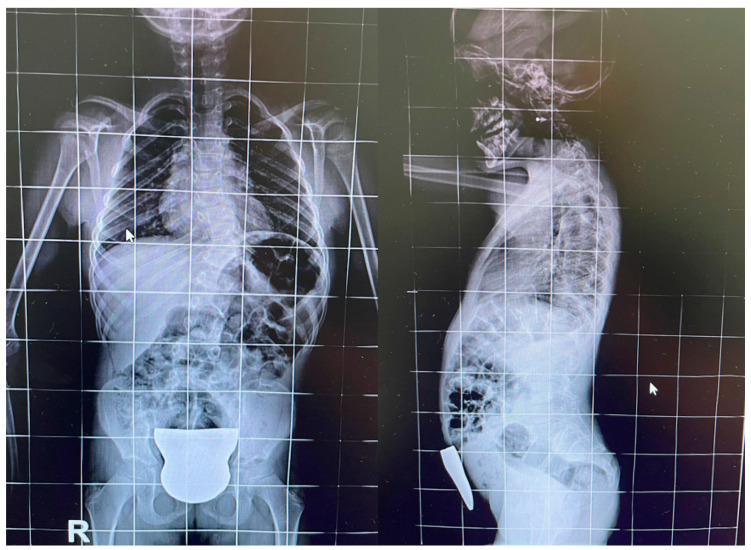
Antero-posterior and lateral X-rays of the patient’s spine.
